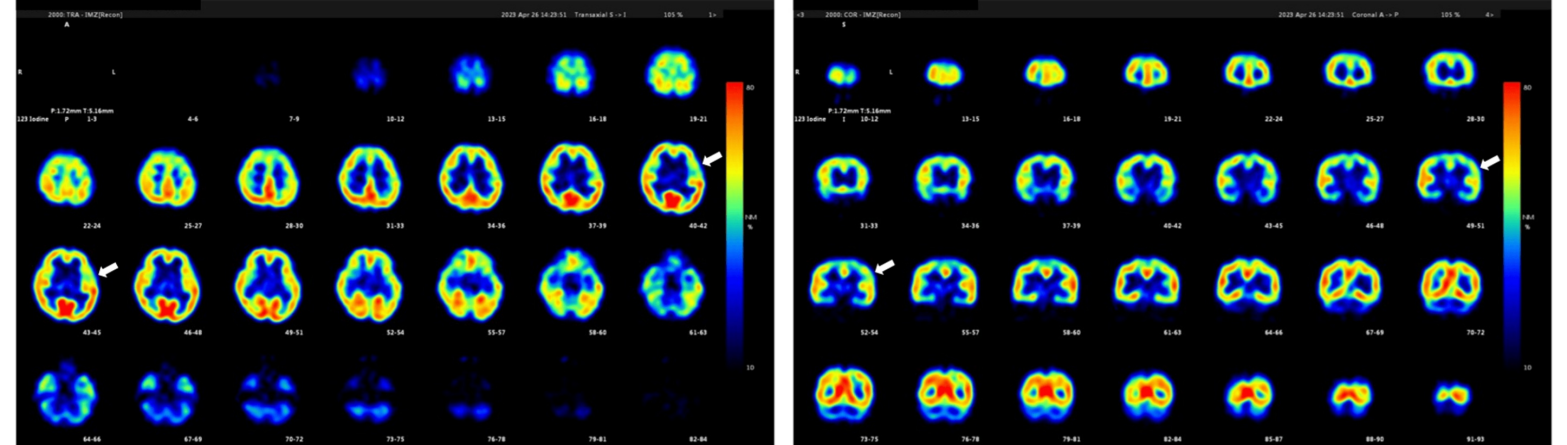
The 123I-IMZ-SPECT reports of the patient Decreased accumulation around the left insular cortex during the interictal period (white arrows) 123I-IMZ-SPECT: iodine-123-iomazenil single-photon emission computed tomography
